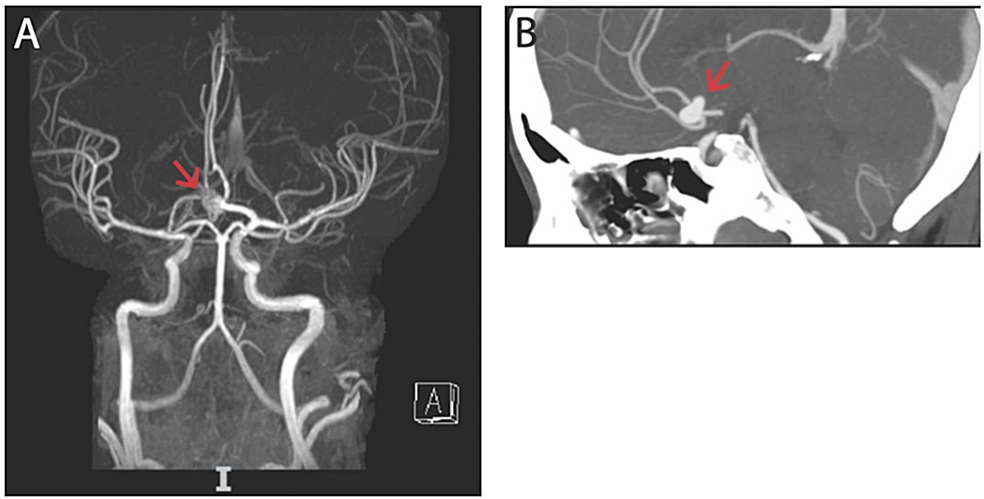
Imaging of patient’s cerebral vasculature A) Magnetic resonance angiogram of the head (coronal view) with intravenous contrast demonstrating stable 10mm saccular aneurysm (arrow) at the junction of the anterior communicating artery and the left A1 segment. The right A1 segment is hypoplastic. B) Computed tomography angiogram of the head demonstrating the same aneurysm in the midsagittal section (arrow).
